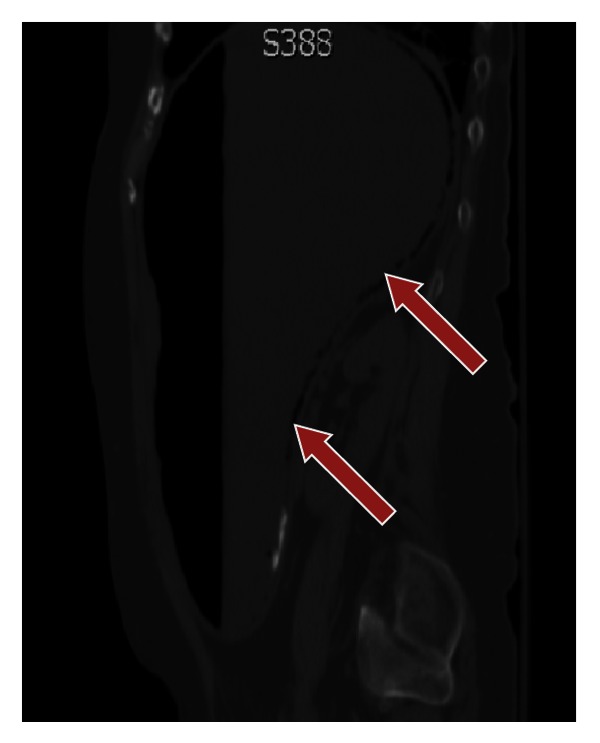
Gastric emphysema better delineated utilizing a bone/lung window on CT scan, sagittal reformat (arrows).
